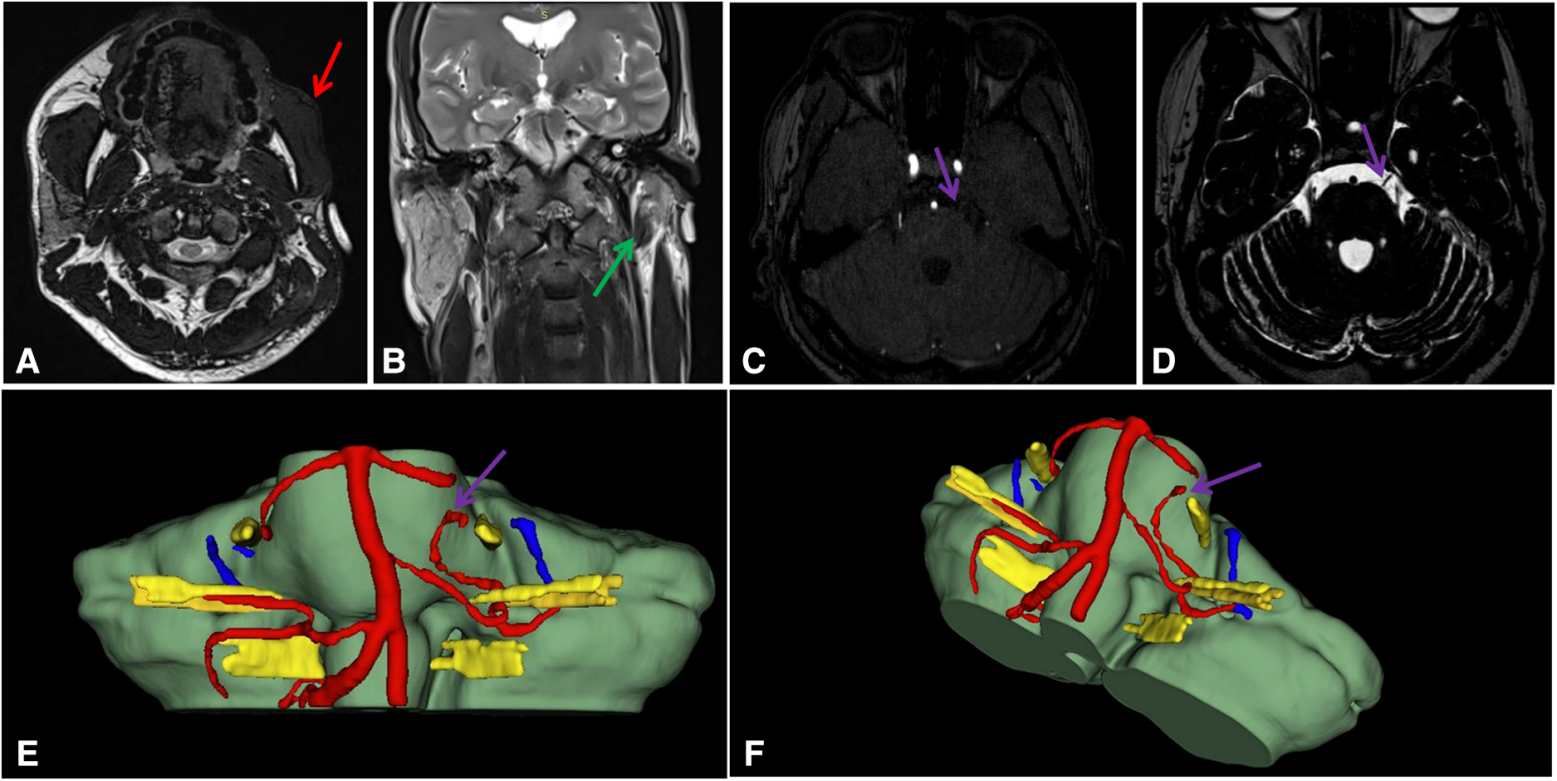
(A–F). Images and 3D reconstruction. (A). Reduction in the subcutaneous fat of the left cheek(Red arrow); (B). Left parotid gland atropy (Green arrow); (C). MRI-3D-TOF; (D). MRI-T2; (E,F). 3D reconstruction. A tiny blood vessel runs ventral to the CN V(Purple arrow).
